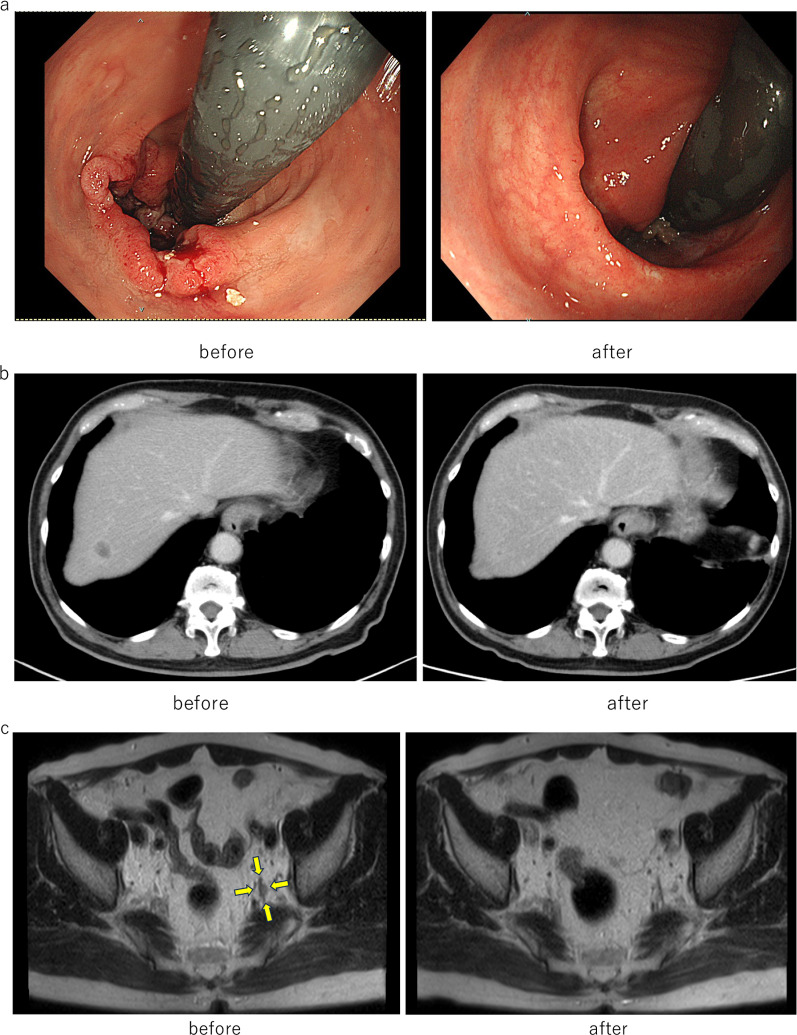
a Shrinkage of rectal cancer with chemotherapy, indicating near complete response, by lower gastrointestinal endoscopy. b Complete response of the single liver metastasis in S6 following chemotherapy. c Magnetic resonance imaging showing suspicious metastasis in lymph node #263L, which has shrunk with chemotherapy and is not detected on imaging
